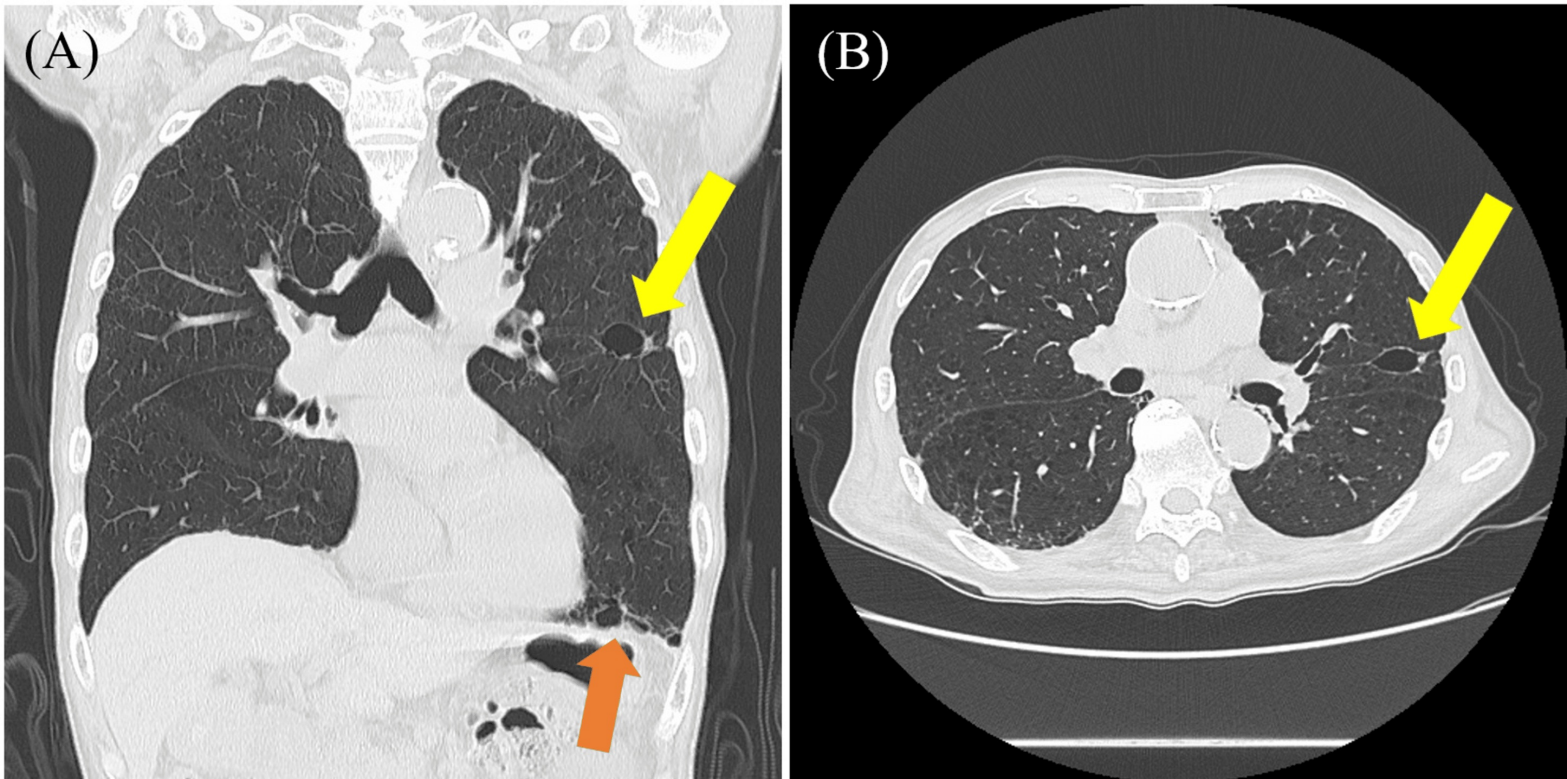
Thoracic computed tomography (CT) scan on lung window. (A, B) Chest CT scan showing pulmonary emphysematous changes (arrows). A: coronal section; B: axial section.
